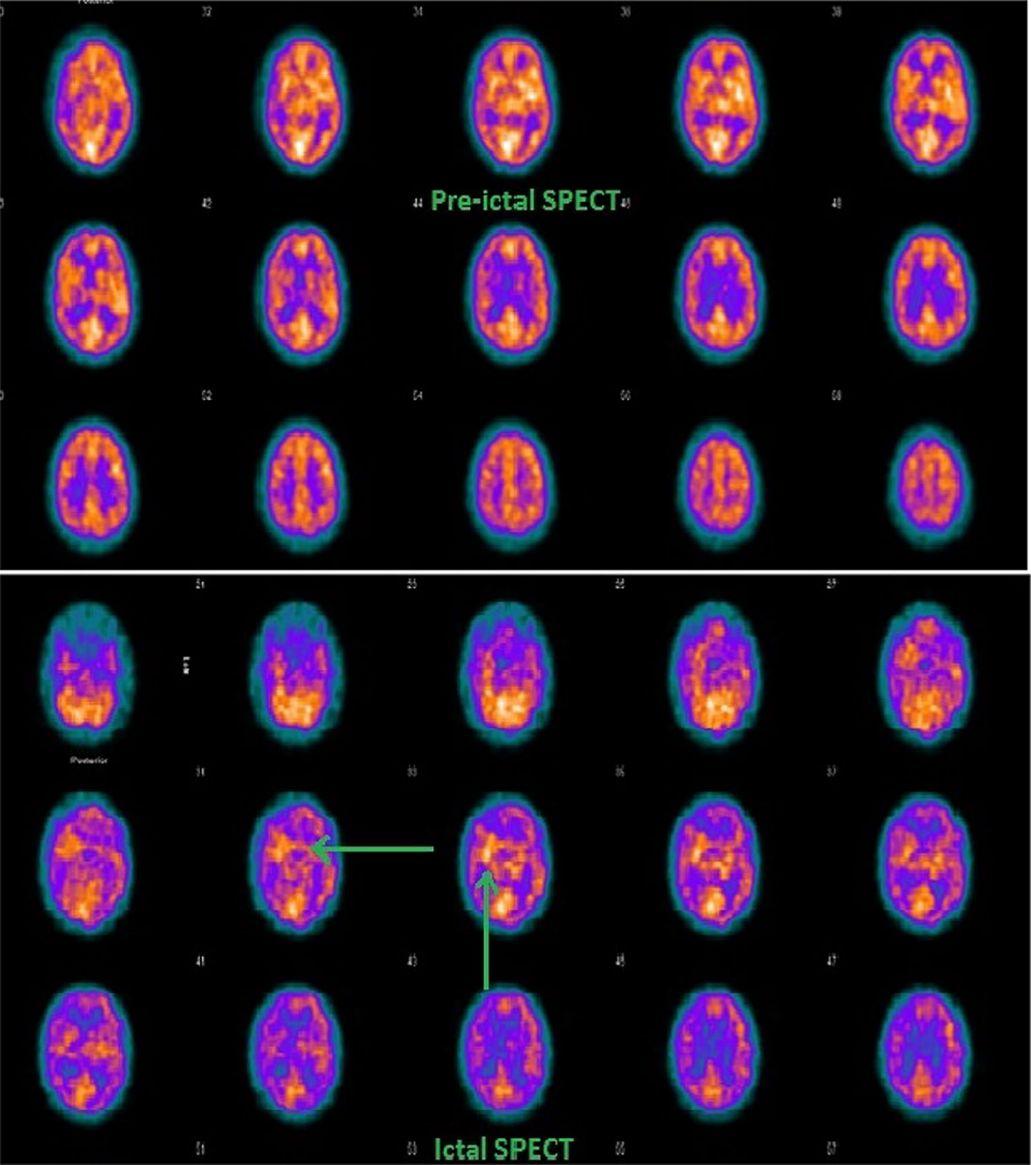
Tc-99 m HMPAO pre-ictal brain SPECT scan (top image) an ictal scan (bottom image) section showing a focal area of hyperperfusion in the right temporal area (green arrows) suggesting a locus of seizure onset here
